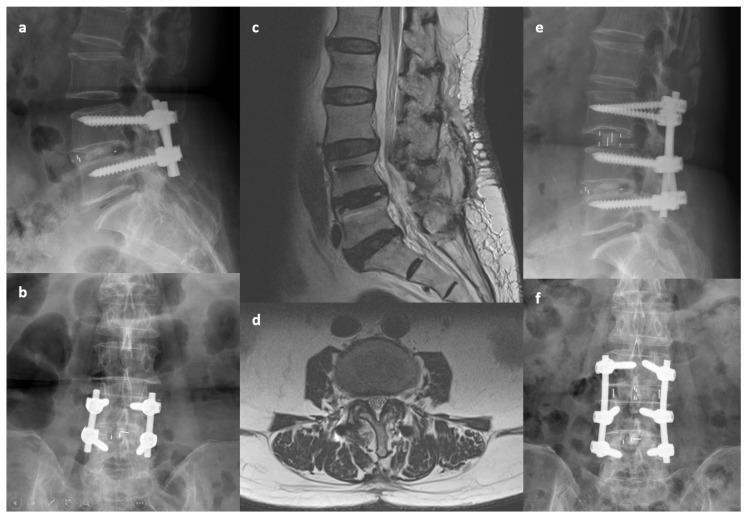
An 81-year-old woman presented with low back pain, left thigh radiation, and claudication for 9 months. She had received transforaminal lumbar interbody fusion performed at the L4-5 segment 7 years previously for spondylolisthesis and spinal stenosis. (a,b) Preoperative X-ray images. (c,d) Preoperative MRI indicating central canal stenosis at L3-4. (e,f) Postoperative X-ray indicating oblique lumbar interbody fusion at L3-4 with extension of posterior instrumentation. The lower endplate of L3 may have been damaged during surgery.
